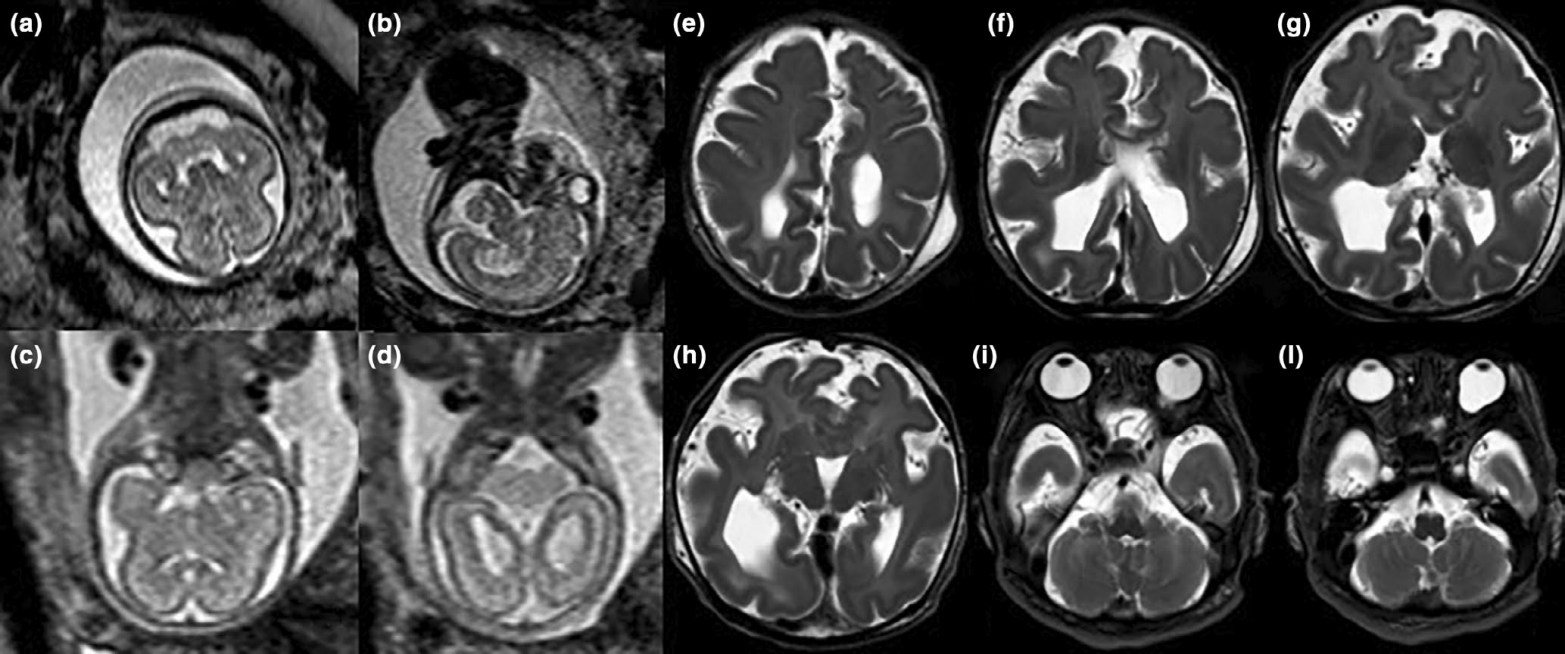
Prenatal diagnosis of lobar holoprosoencephaly. The baby was born alive but developed intractable epilepsy and died in a few days. (a–l) Fetal magnetic resonance imaging (MRI) (20 weeks of gestational age) (a–d) and postnatal cerebral MRI (e–l) showed absent anterior third of the corpus callosum, absent pellucid septum, unseparated frontal lobes, diffuse irregular and abnormal cortical profile, biparietal and fronto‐occipital cerebral diameter reduction.
